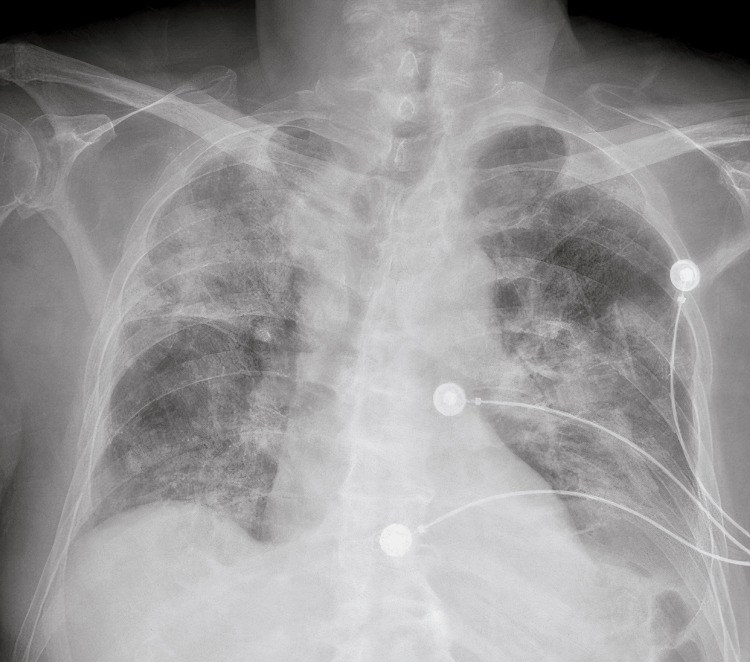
Chest x-ray (admission): increased opacity in the lower left side of the chest (hemithorax), consistent with either a pleural effusion or a pulmonary infiltrate.
